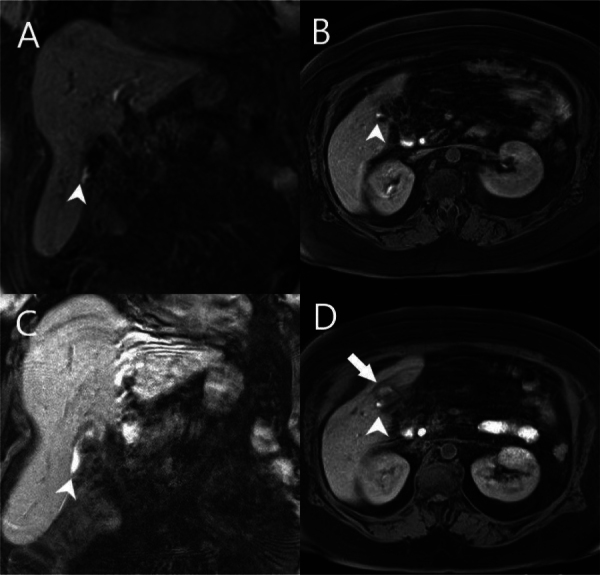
Gd-EOB-DTPA-enhanced T1-weighted 3D GRE images at 50 minutes (A: coronal, B: axial) and 2 hours (C: coronal, D: axial) post-injection. The subvesical bile duct (arrowhead) supplies the biloma with hepatobiliary contrast-enhanced bile (arrow).
